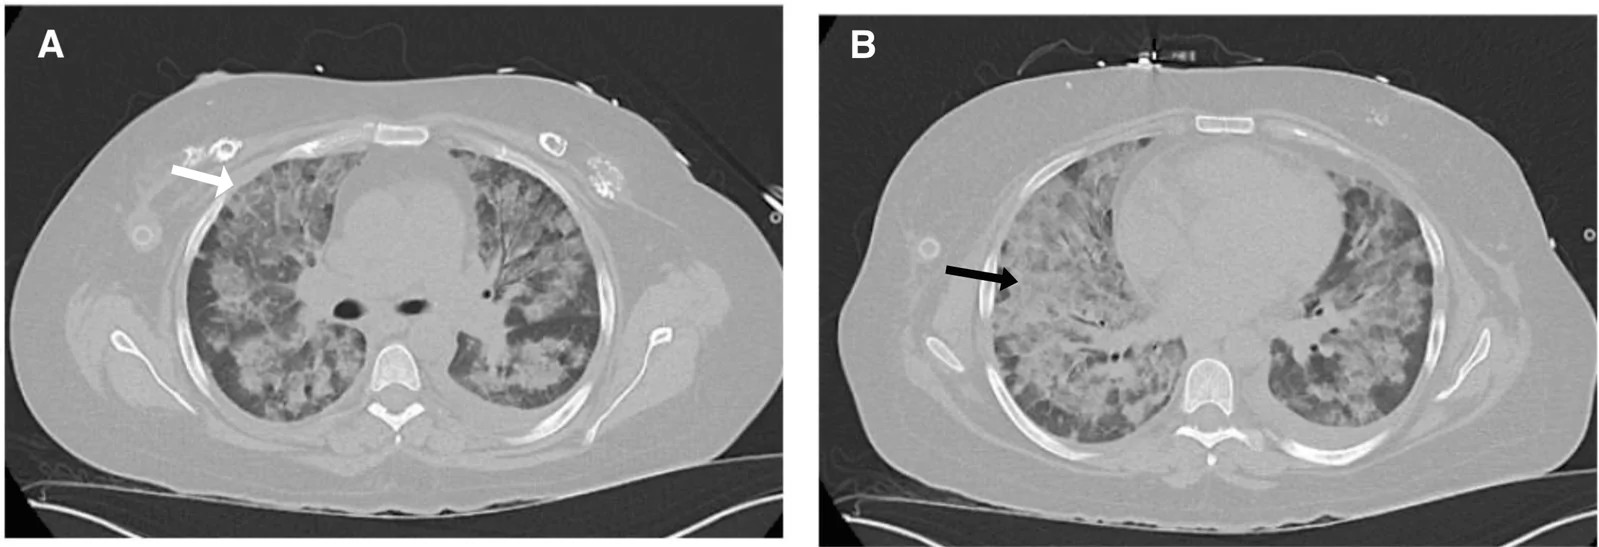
Non-contrast chest CT obtained one day after repeat chest radiography demonstrated (a) diffuse bilateral ground-glass (white arrow) and (b) consolidative changes (black arrow) throughout both lungs.
